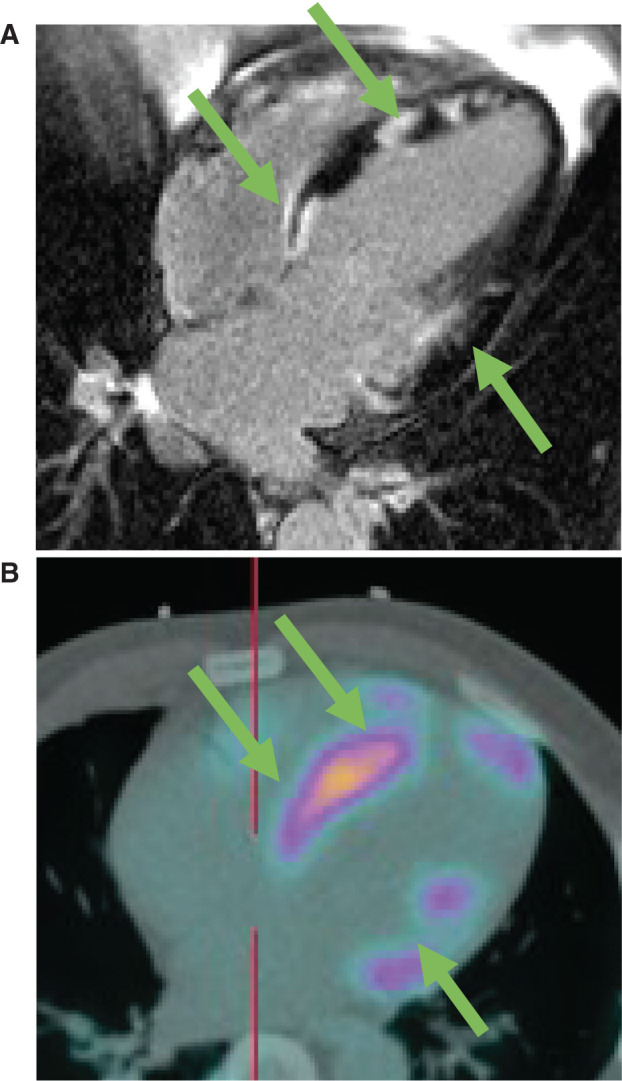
A: Delayed hyperenhancement of a patient with cardiac sarcoidosis demonstrates diffuse, patchy involvement in areas throughout the right and left ventricles (arrows). B: FDG-PET demonstrates active inflammation in areas that correspond to areas of LGE. Courtesy of Steven R. Sigman, MD.
